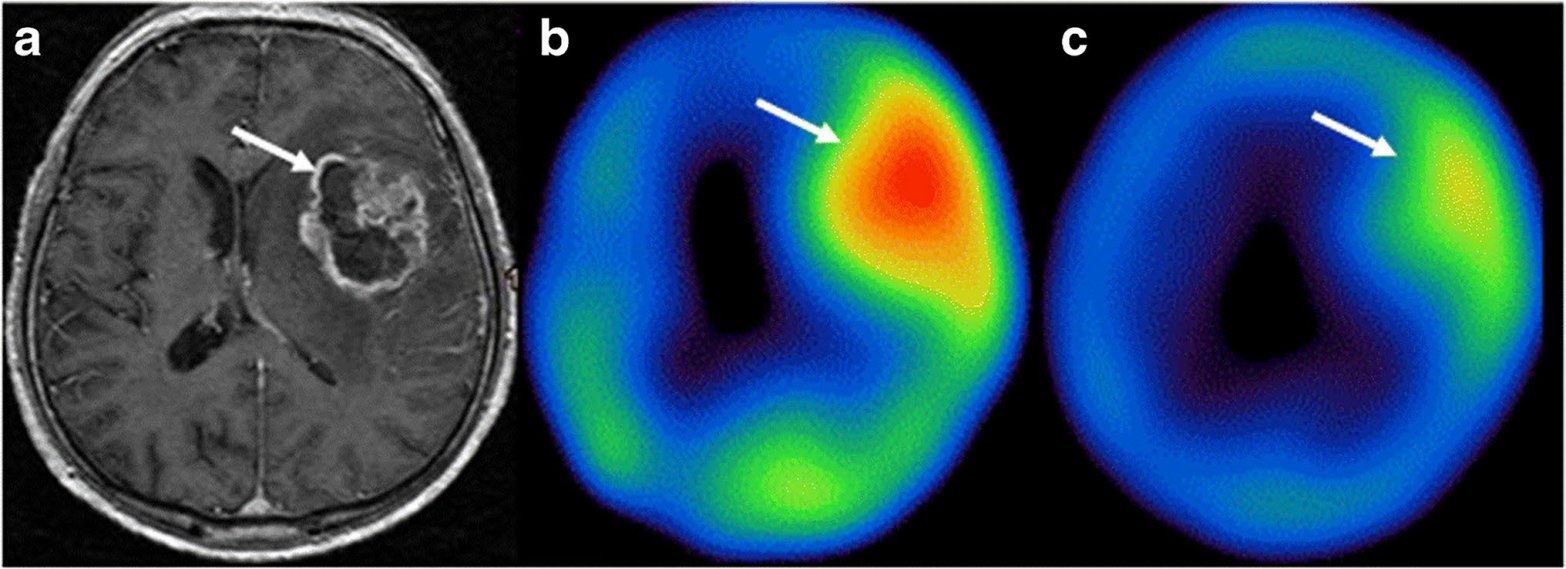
[^123^I]I-VEGF_165_ SPECT/MRI of a grade IV glioma in a 60-year-old patient. a Acial T1-weighted MRI for anatomical reference of the tumor lesion. b [^123^I]I-VEGF SPECT imaging before radiation therapy and c lower radioligand accumulation 1 week post-radiation therapy. White arrows indicate the tumor lesion. The figure was originally published in EJNMMI by Rainer et al. [163]. The prognostic value of [^123^I]-vascular endothelial growth factor ([.^123^I]-VEGF) in glioma (2018) Vol. 45(13): 2396–2403
